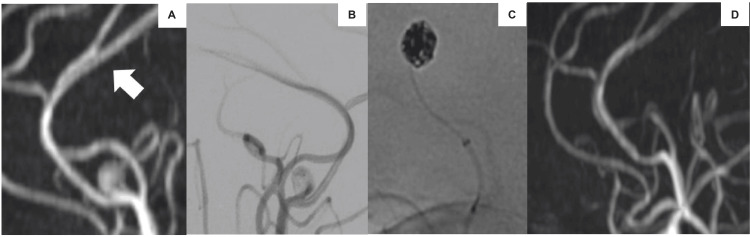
Re-enlargement of aneurysm. (A) MRI one year after onset reveals enlargement of the aneurysm of the left pericallosal artery and disappearance of the other aneurysm (arrow). (B, C) Coil embolization was performed. (D) MRI six months postoperatively. (A), (B), and (D) - lateral view.
